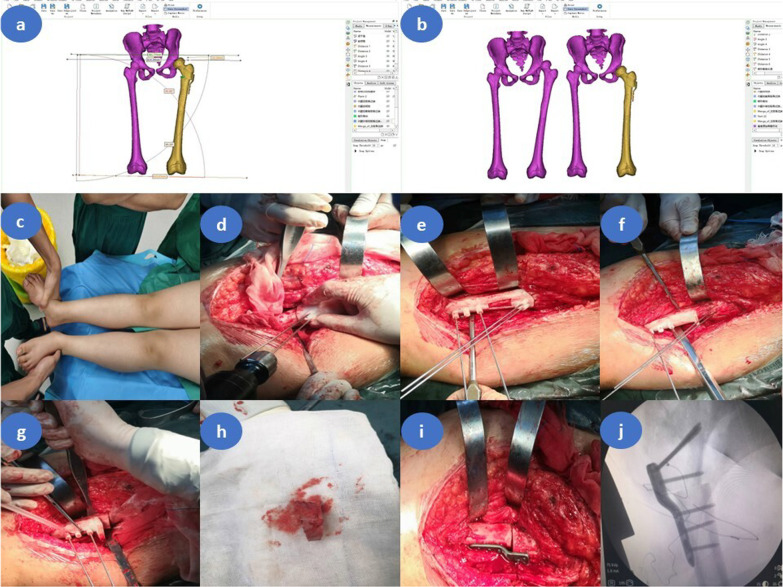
Intraoperative application of the osteotomy navigation templates in children with left developmental dysplasia of the hip (female patient, 5.5 years old, Tönnis type III). a Preoperative surgical planning in 3-Matic software. b Comparison of preoperative and postoperative CT scan three-dimensional reconstruction in 3-Matic software. c Both lower limbs are unequal, with the left shorter than the right. d Kirschner wires placement assisted by the positioning guide. e The distal Kirschner wires were properly inserted with the assistance of the connecting guide plate. f Implantation of the oblique osteotomy guide plate under the guidance of the distal Kirschner wires. The angle tilted cephalad to the osteotomy guide is the angle of varus of the proximal femur, and the first osteotomy was conducted by the swing saw with the aid of the guide. g The transverse osteotomy guide plate was placed under the guidance of distal Kirschner wires. The osteotomy surface of the guide plate was perpendicular to the femur, and the second osteotomy was conducted by the swing saw with the aid of the guide plate. The angle between the two osteotomy surfaces is the proximal femoral varus angle, and the length of the lateral femoral cortex between the two osteotomy surfaces is the additional shortening distance of the femur. h Trapezoidal bone block cutoff with the assistance of the guide plates. i LCP-PHP fixation of the distal and proximal femur. j C-arm fluoroscopy showed the plate was well-positioned and the anticipated orthopedic result was achieved
